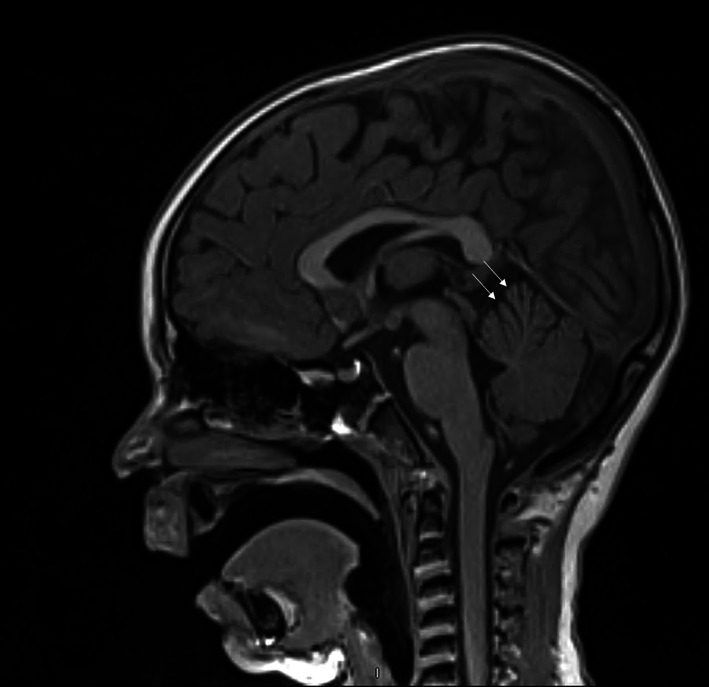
Sagittal T1 brain MRI shows mild atrophy of the anterior lobe of the cerebellum.
